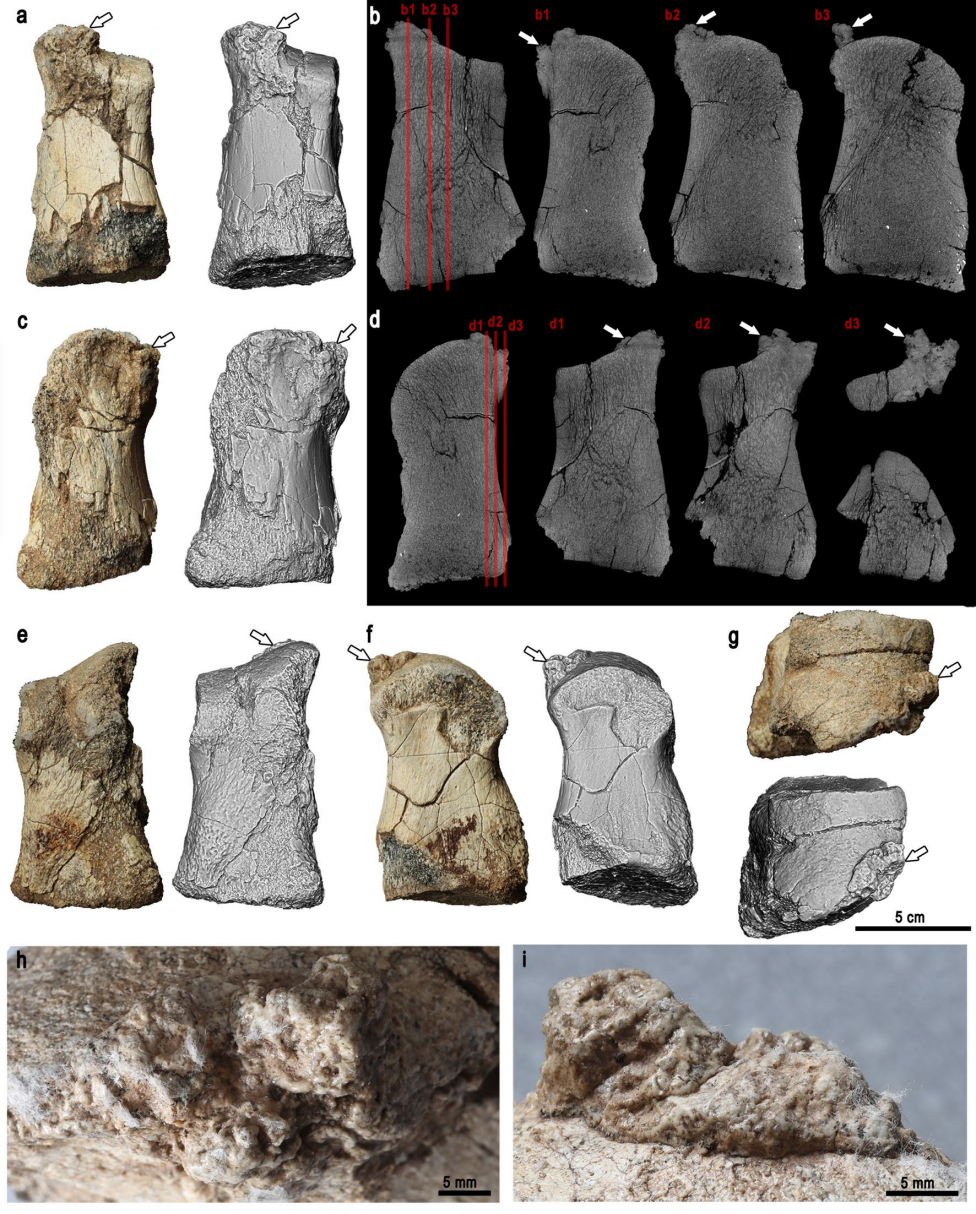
Phalanx 1 of digit IV. (a) Dorsal view. (b) Dorsoventral CT sections, planes of sectioning indicated by red lines on the lateromedial CT section. (c) Lateral view. (d) Lateromedial CT sections, planes of sectioning indicated by red lines on the dorsoventral CT section. (e) Ventral view. (f) Medial view. (g) Distal view. White arrows indicate the calcium deposit. (h–i) Closeups of the calcium deposit.
